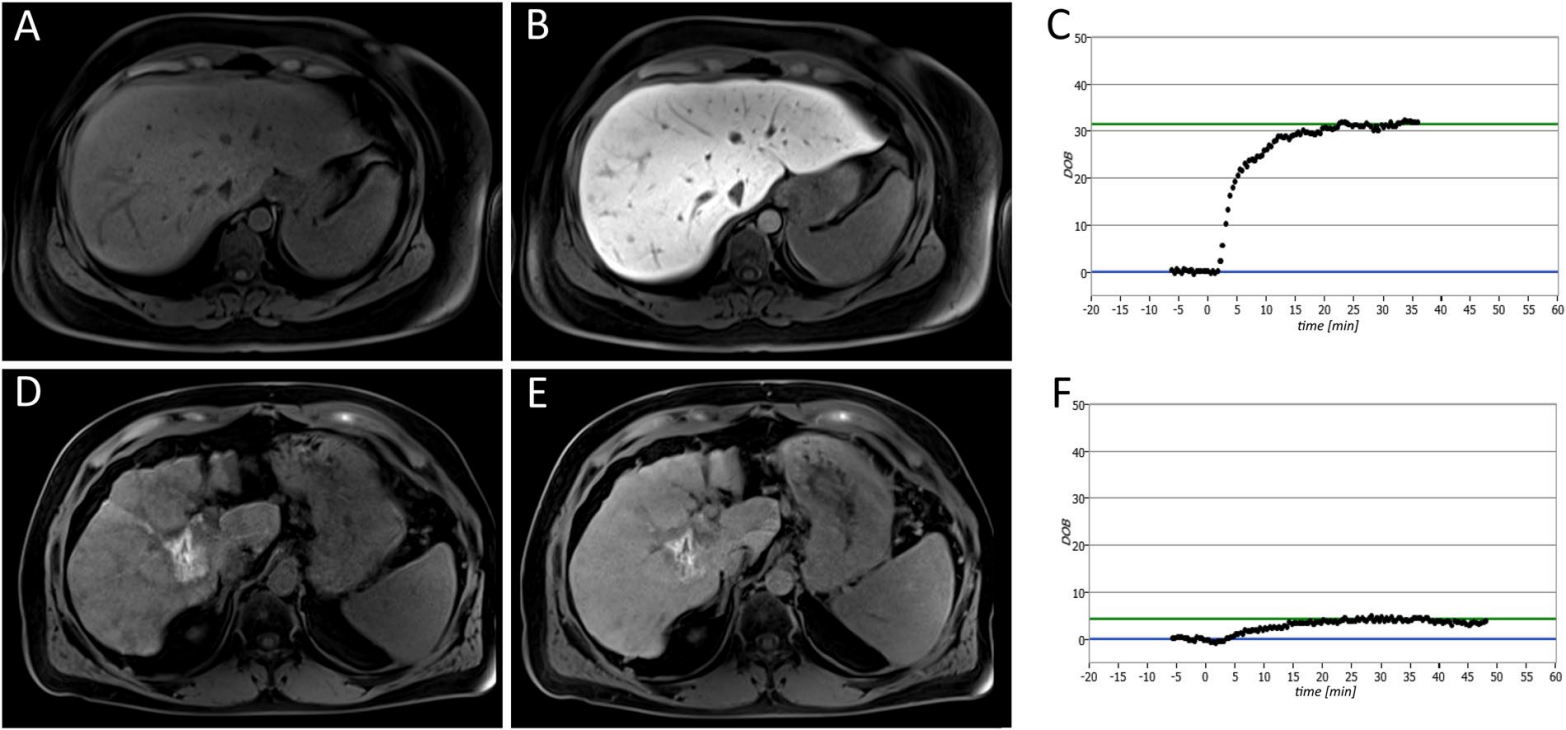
Liver function analysis in patients with normal liver function (A,B,C) and severely impaired liver function (D,E,F). The patients underwent plain (A,D) and Gd-EOB-DTPA-enhanced HBP (B,E) T1-weighted VIBE sequences with fat suppression, as well as a ^13^C-MBT, over a maximum time span of 60 min (C,F). In the case of the displayed patients, a ^13^C-MBT readout value of 409 µg/kg/h (C) was considered normal liver function (SI_pre_, 182.67; SI_post_, 441.67; RE, 1.42), while a ^13^C-MBT readout value of 57 µg/kg/h (F) was considered impaired liver function (SI_pre_, 258.50; SI_post_, 332.83; RE, 0.29). The lesion observed in the liver with impaired function (D,E) was caused by former radiofrequency ablations treatments. The delta-over-baseline (DOB) was assessed inline automatically and describes the increase in the R_PDB_-corrected ^13^CO_2_:^12^CO_2_ ratio to the basal value (blue line). The evaluated ^13^C-MBT value was calculated as the product of the DOB_max_, R_PDB_, CO_2_ production and molar mass of ^13^C-methacetin per body weight^13^. The DOB_max_ (green line) was defined after an increase in DOB was no longer observable. At the time point 0, the ^13^C-methacetin was applied via bolus injection.
